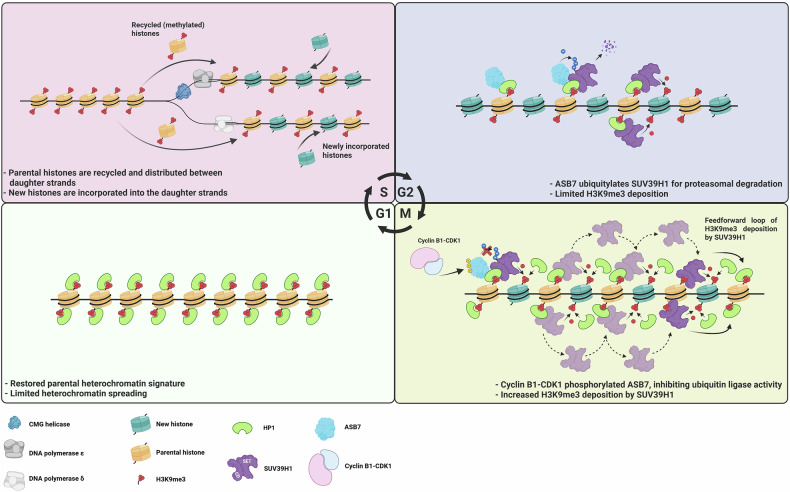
The HP1–SUV39H1–ASB7 regulatory loop orchestrates H3K9me3 homeostasis across the cell cycle. During S-phase (top left), parental H3K9me3-marked histones are redistributed between daughter strands, and incorporation of newly synthesized histones leads to dilution of the repressive mark. To counteract excessive H3K9me3 spreading driven by the HP1–SUV39H1 positive-feedback loop (top right), ASB7 ubiquitylates chromatin-bound SUV39H1, targeting it for proteasomal degradation and thereby restricting its invasion into euchromatic regions. In mitosis (bottom right), CDK1–Cyclin B1 phosphorylates ASB7, transiently inhibiting its E3 ligase activity and permitting SUV39H1 accumulation and H3K9me3 re-establishment on new histones. Following mitosis (bottom left), ASB7 is reactivated to restrict further spreading, thereby ensuring restoration and maintenance of heterochromatin identity across cell divisions. Created in Biorender (https://BioRender.com)
